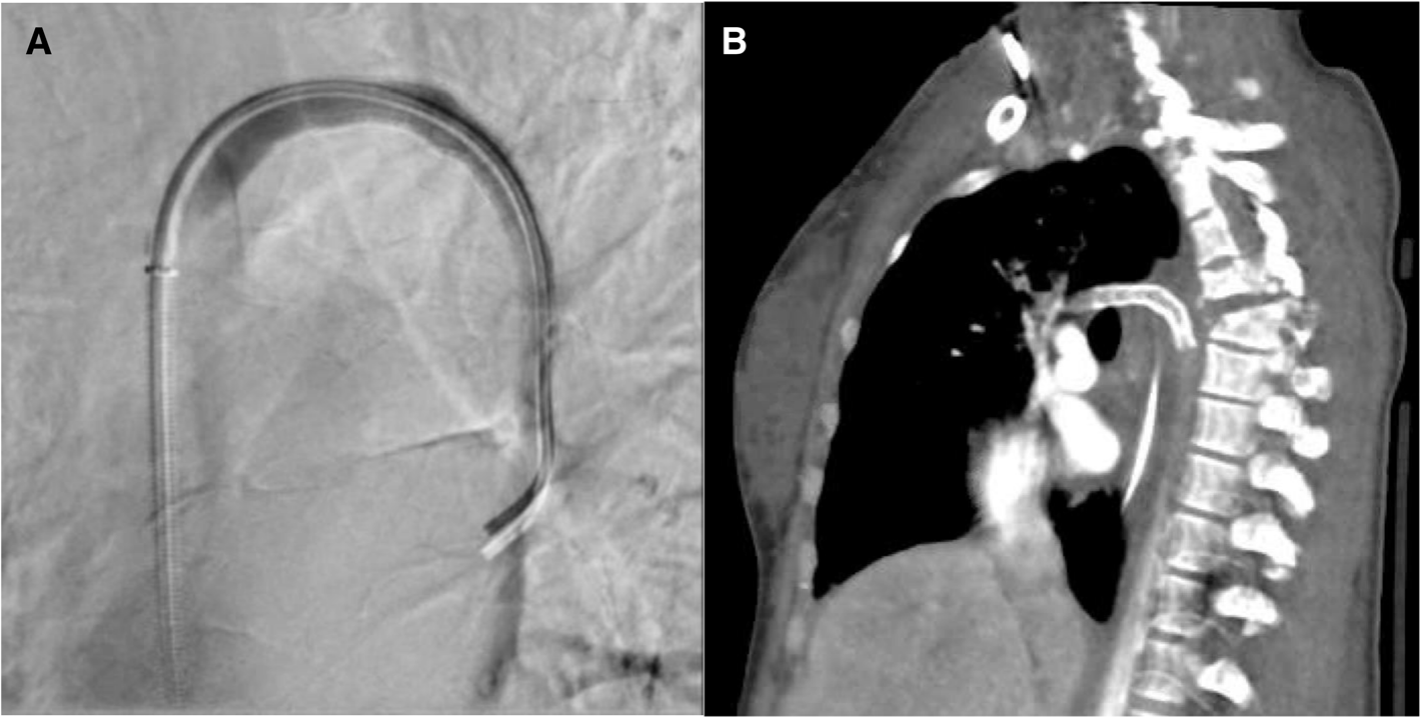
A Post procedural DSA confirms no extravasation after Viabahn stent graft placement. B CT (sagittal oblique view) performed on post-procedure day eight, though suboptimally timed for venous evaluation, shows no evidence of hematoma, hemorrhage, or other stent malfunction
